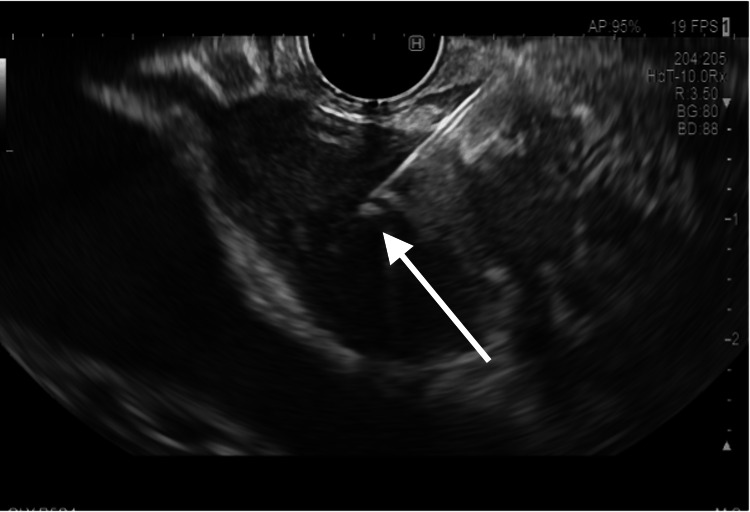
Hypoechoic lesion in the pancreatic head on endoscopic ultrasound (EUS) EUS image demonstrating a hypoechoic lesion in the head of the pancreas, without a visible cystic component. Fine needle aspiration was performed for microbiological analysis. Arrows indicate the hypoechoic lesion.
